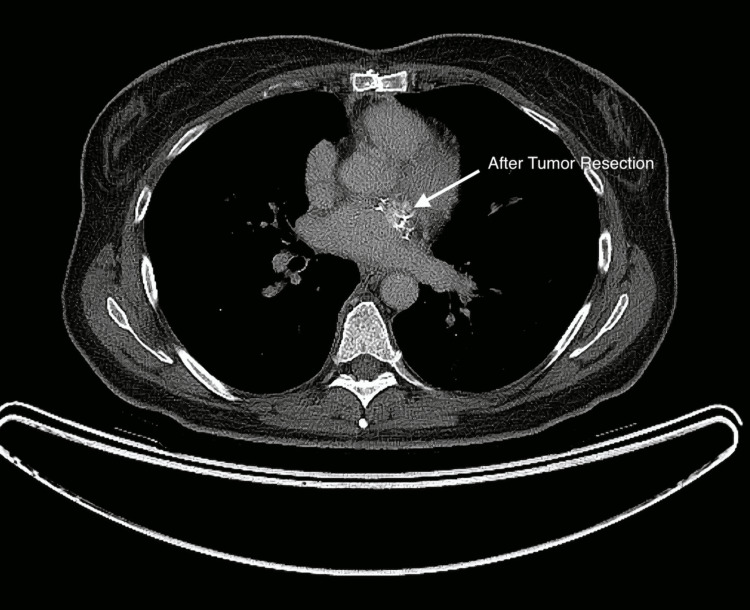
Postoperative CT scan on 12/27/2017 demonstrating no residual disease.
